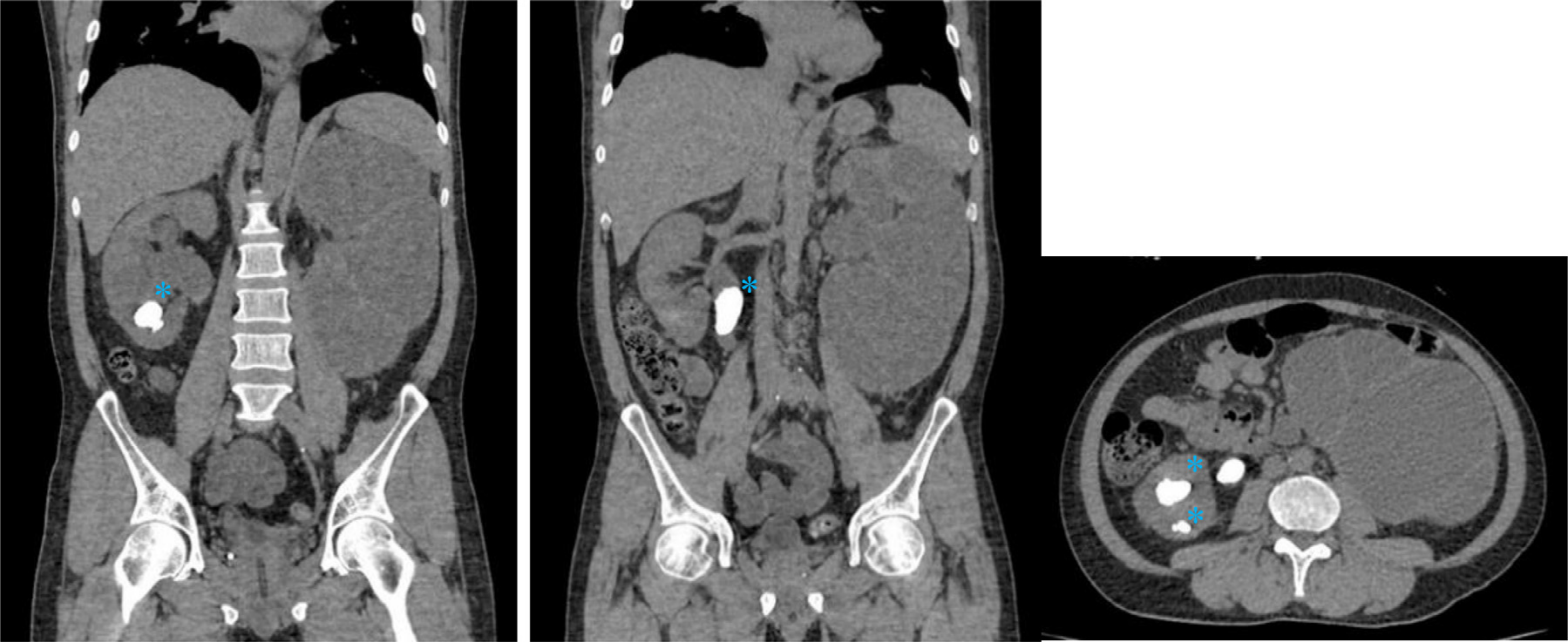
CT scan images depicted the stones (Asterix) at the proximal right ureter, causing dilatation of the structures above. Right hydronephrosis equivalent to 2nd – 3rd grade and right hydroureter were also visible.
